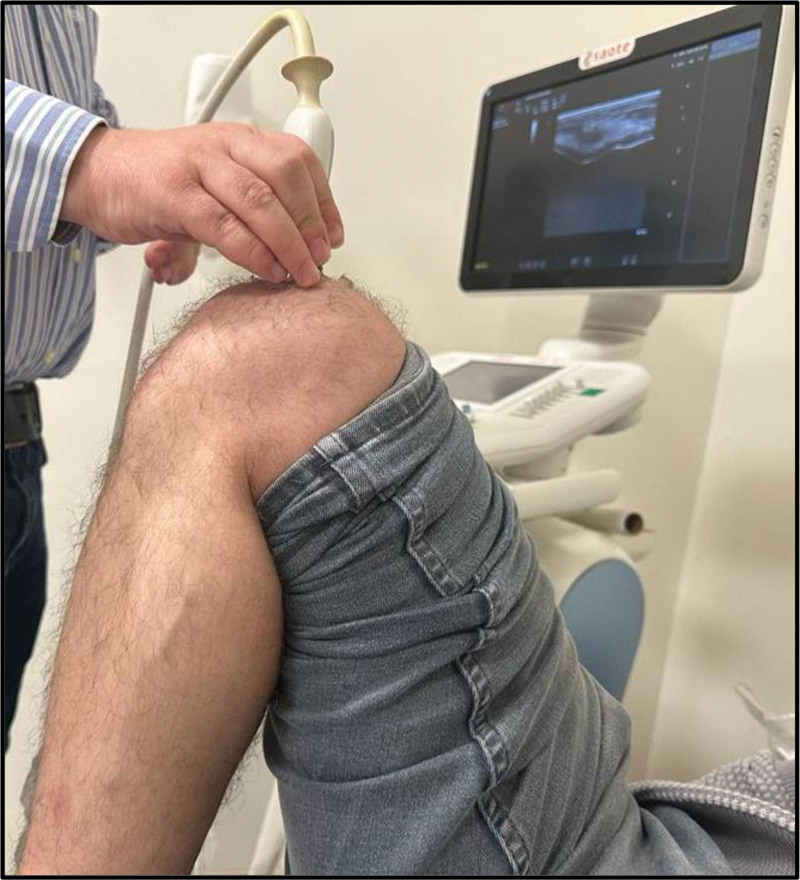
Position of the probe and the patient during ultrasonographic evaluation.
